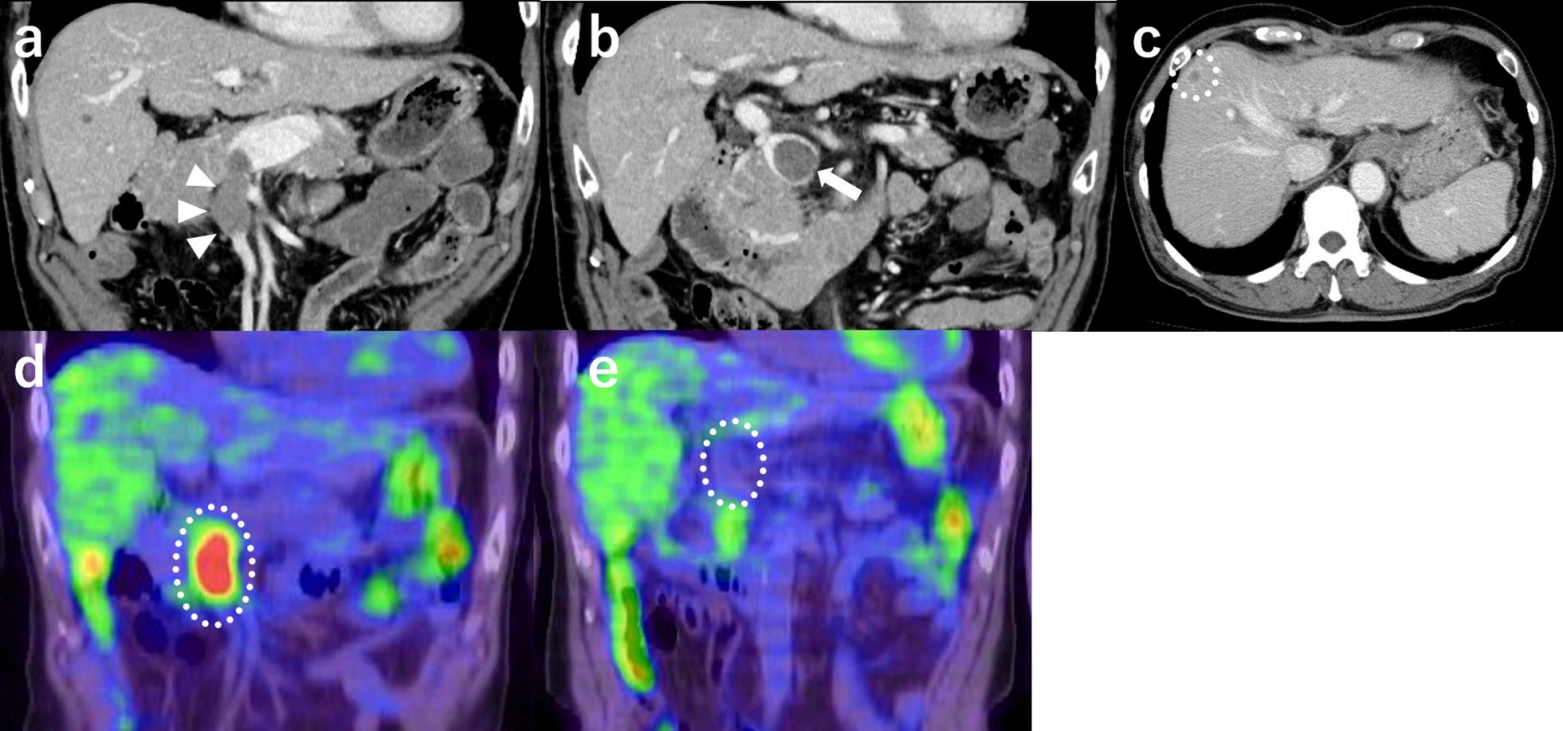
Computed tomography (CT) and positron emission tomography (PET)–CT images. CT showing tumor thrombosis in the superior mesenteric vein (SMV; a arrowhead), portal vein thrombosis (b arrow), and liver metastasis (c white dotted line). On PET–CT, the tumor thrombus in the SMV demonstrated fludeoxyglucose uptake (d white dotted line), but the portal vein thrombus did not (e white dotted line)
